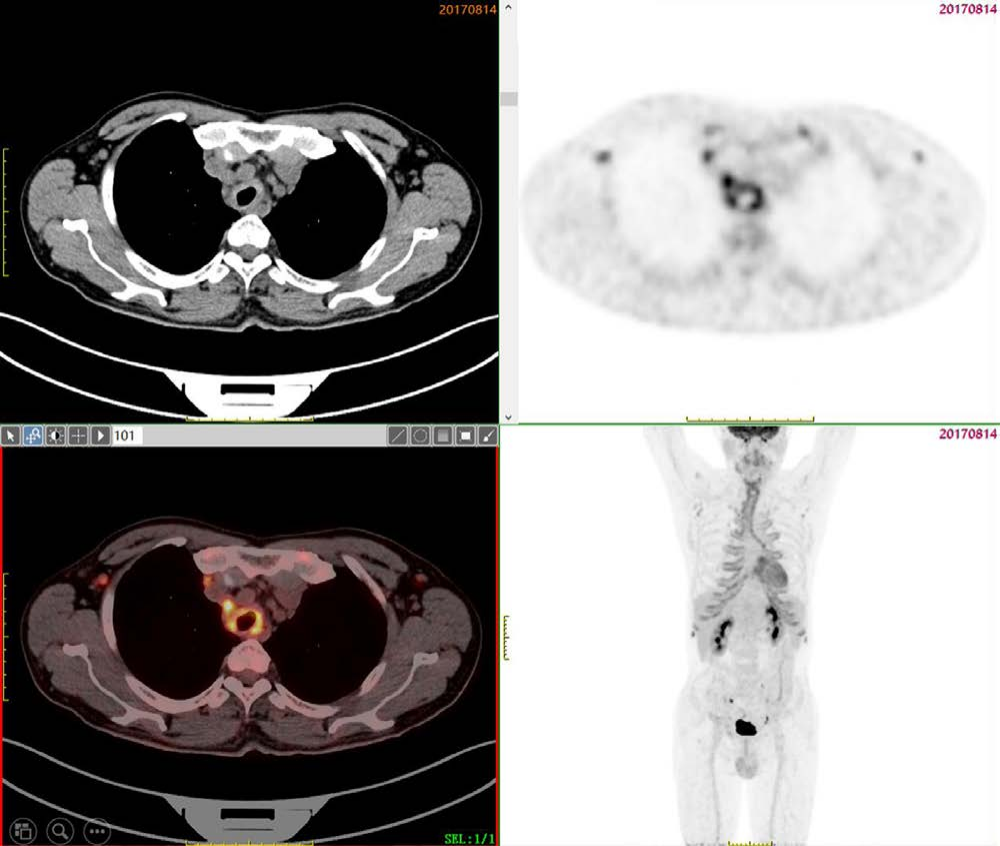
PET-CT demonstrated abnormal metabolic activity in the thyroid cartilage, tracheal cartilage, and bilateral rib cartilage. PET-CT = positron emission tomography–computed tomography.
